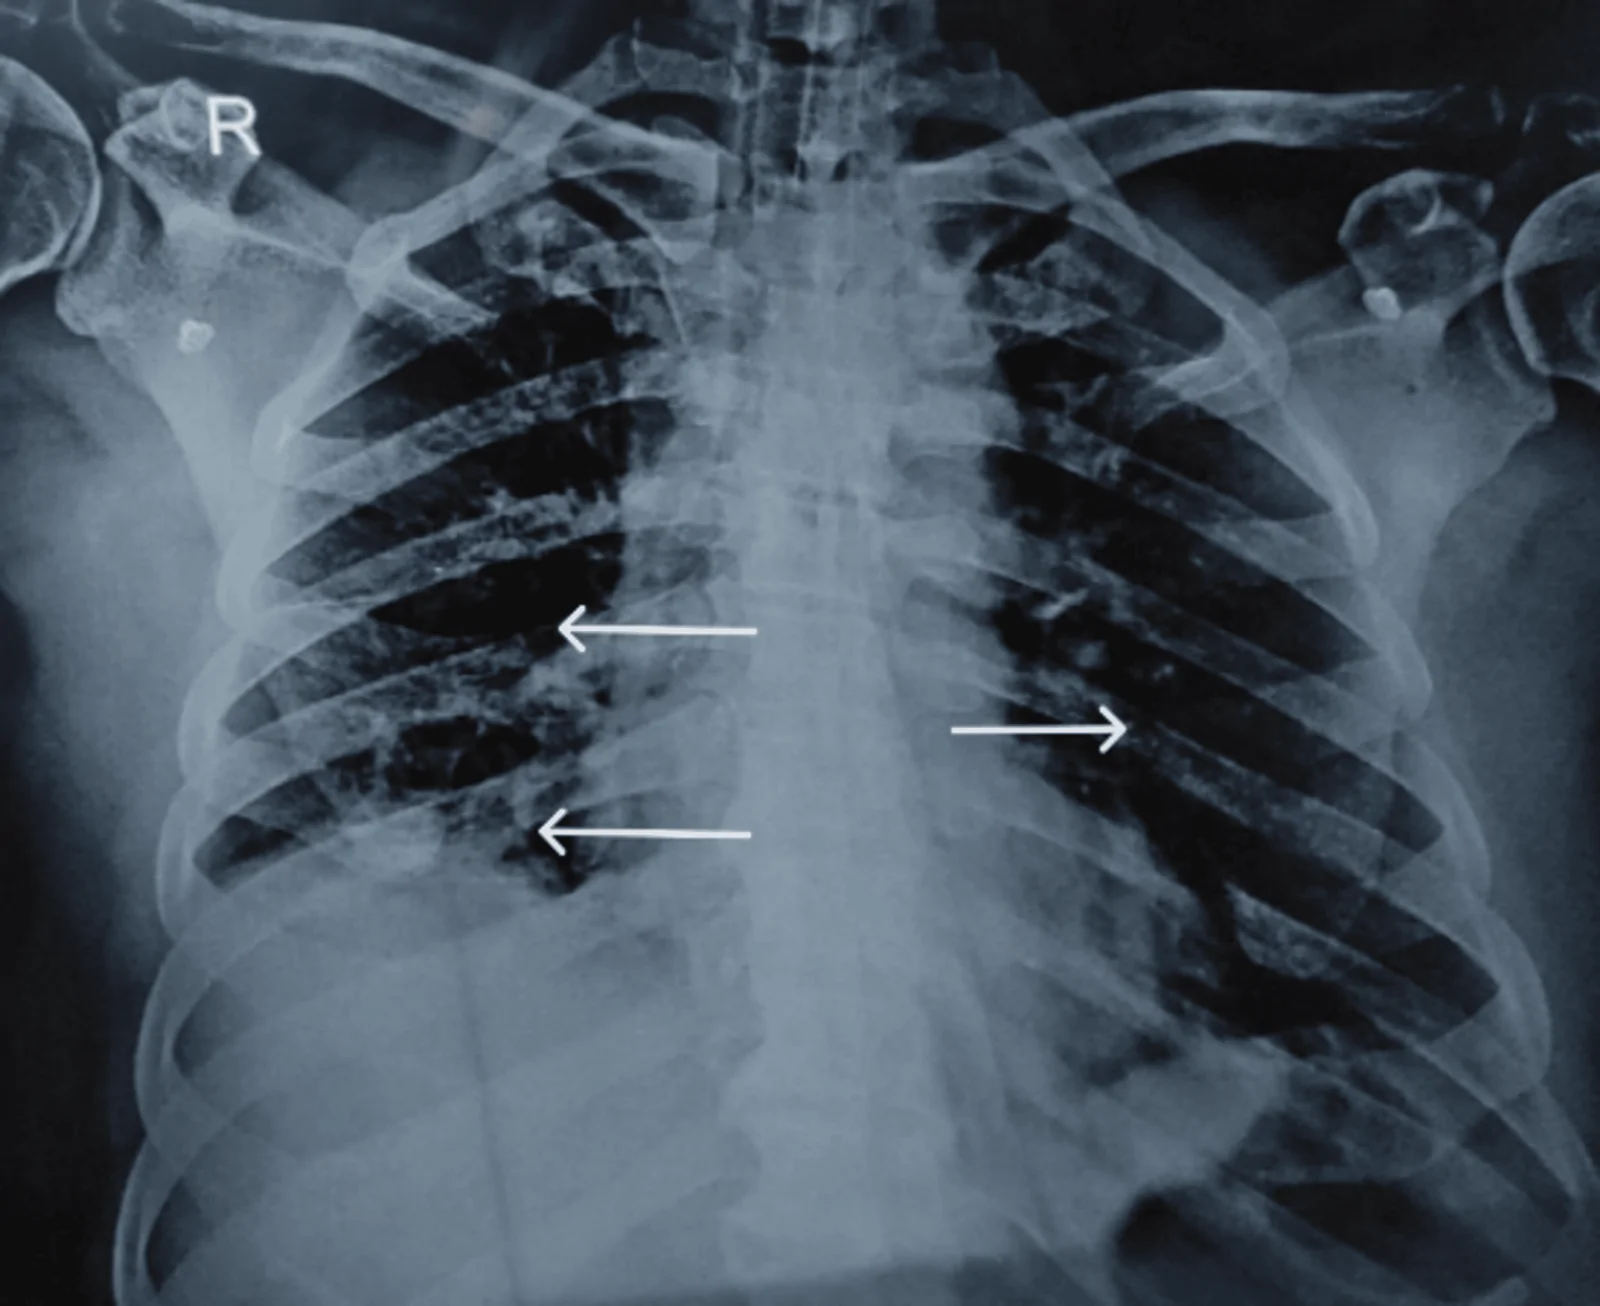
Chest radiograph demonstrating bilateral patchy air-space opacities White arrows indicate bilateral patchy air-space opacities, consistent with multifocal pulmonary infiltrates. Right lower lobe consolidation is also evident
